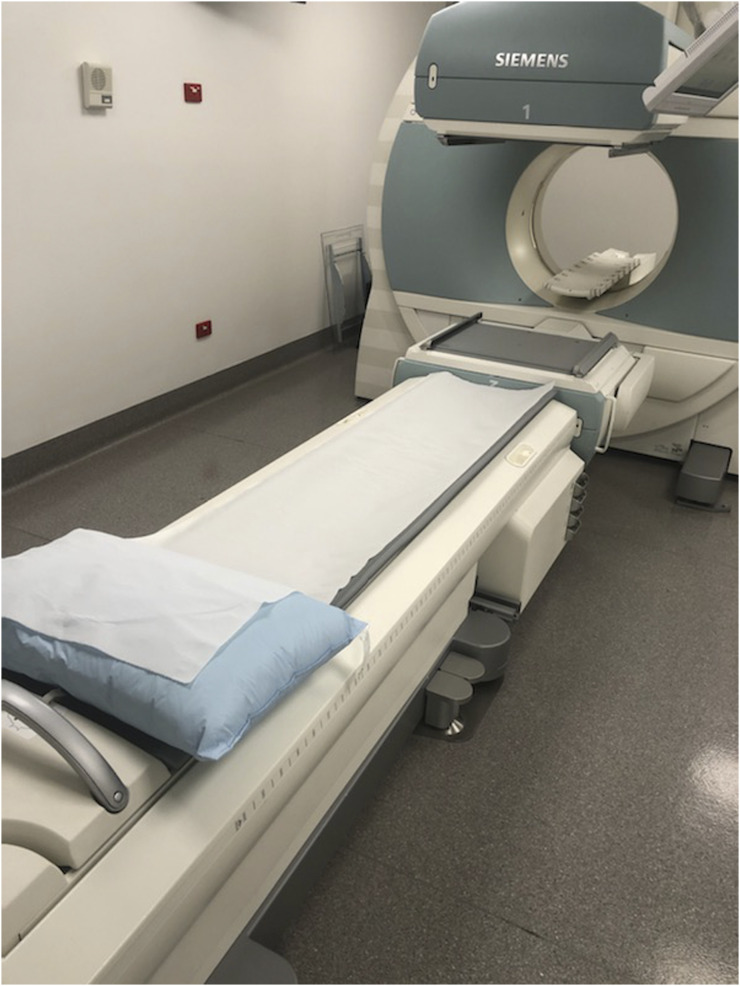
New normal for γ-camera suite, with removal of linen and replacement with single-use paper roll. Room is decluttered for decreased risk and easy cleaning between patients. (Courtesy of Queensland Xray.)
